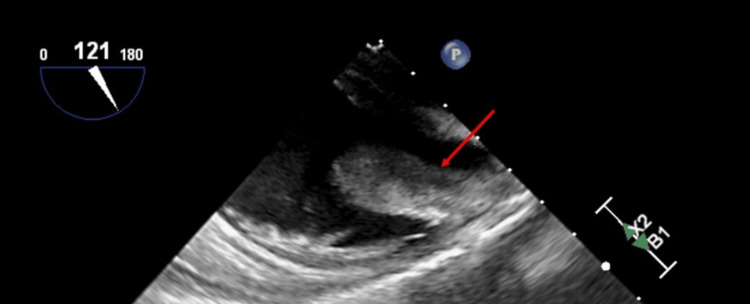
Intraoperative transesophageal echocardiogram (TEE). Mid-esophageal bicaval view demonstrating a 3.2 cm × 1.13 cm echogenic mass (red arrow) anchored at the junction of the superior vena cava (SVC) and right atrium (RA).
